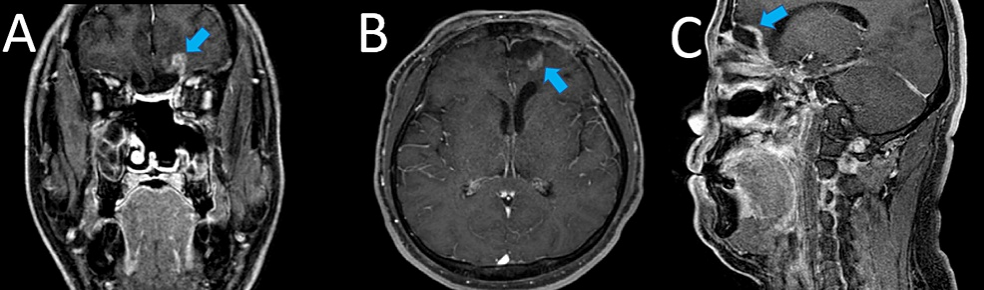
Contrast-enhanced MRI 13 months after the first treatment Coronal (A), axial (B), and sagittal (C) planes of MRI showed enhanced lesions in the left frontal lobe (blue arrow).
